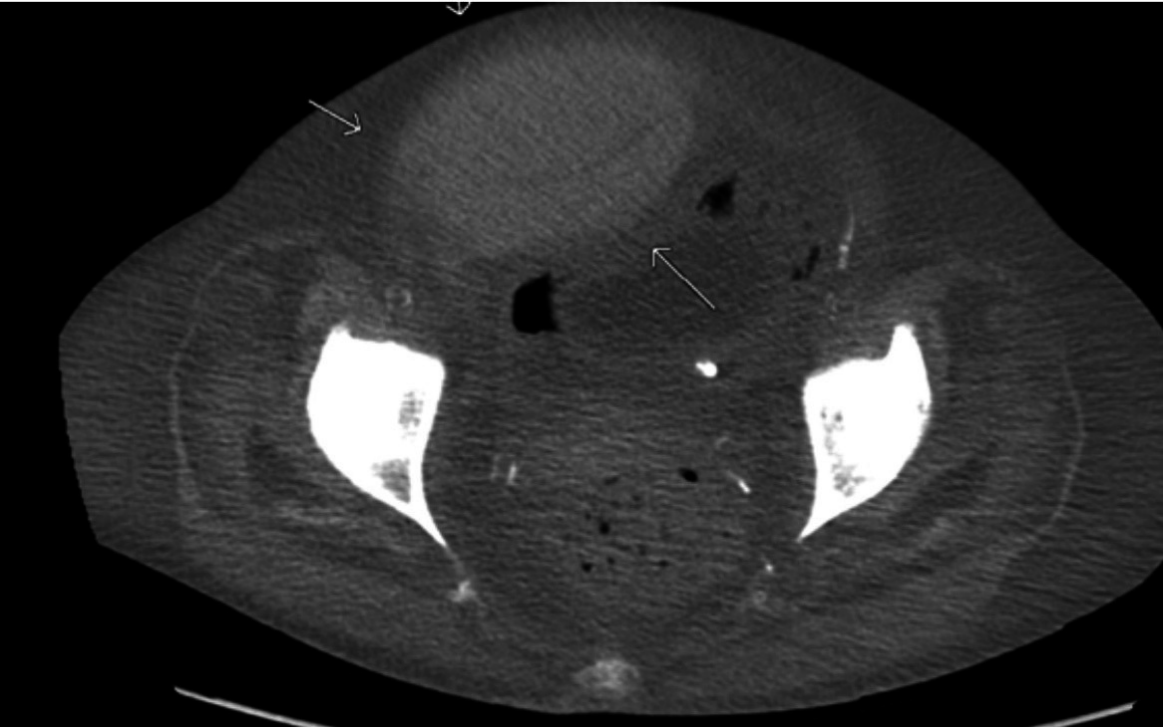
Computed tomography (CT) scan of abdomen and pelvis without contrast showing a 12.6 cm × 9.3 cm × 5.6 cm large mass along the anterior lower abdominal wall representing a bleed in the rectus muscle.
